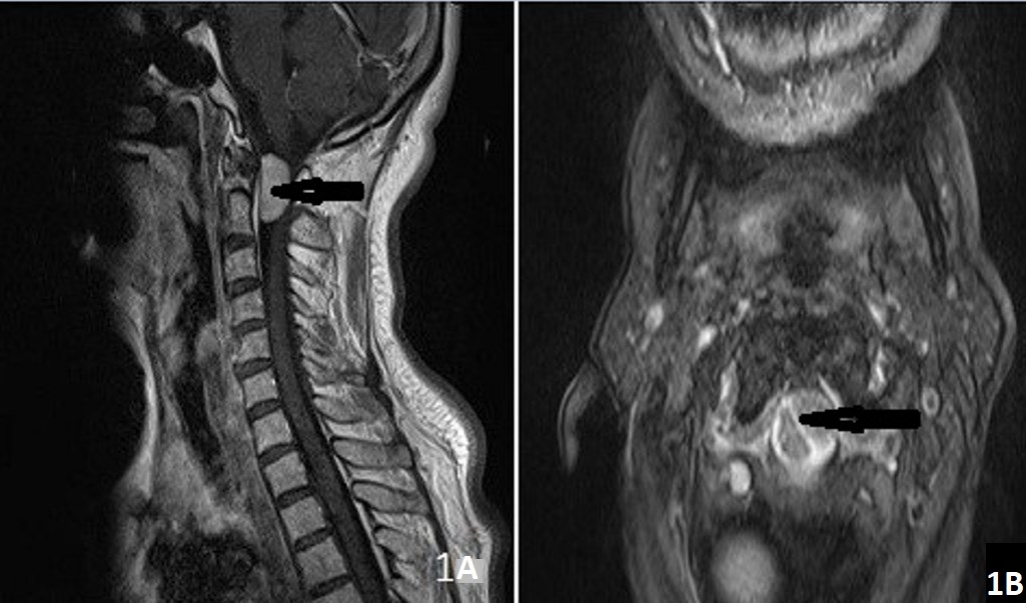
62 years old female patient (A) pre-operative sagittal MRI, (B) pre-operative axial MRI. Black arrow indicates tumor
